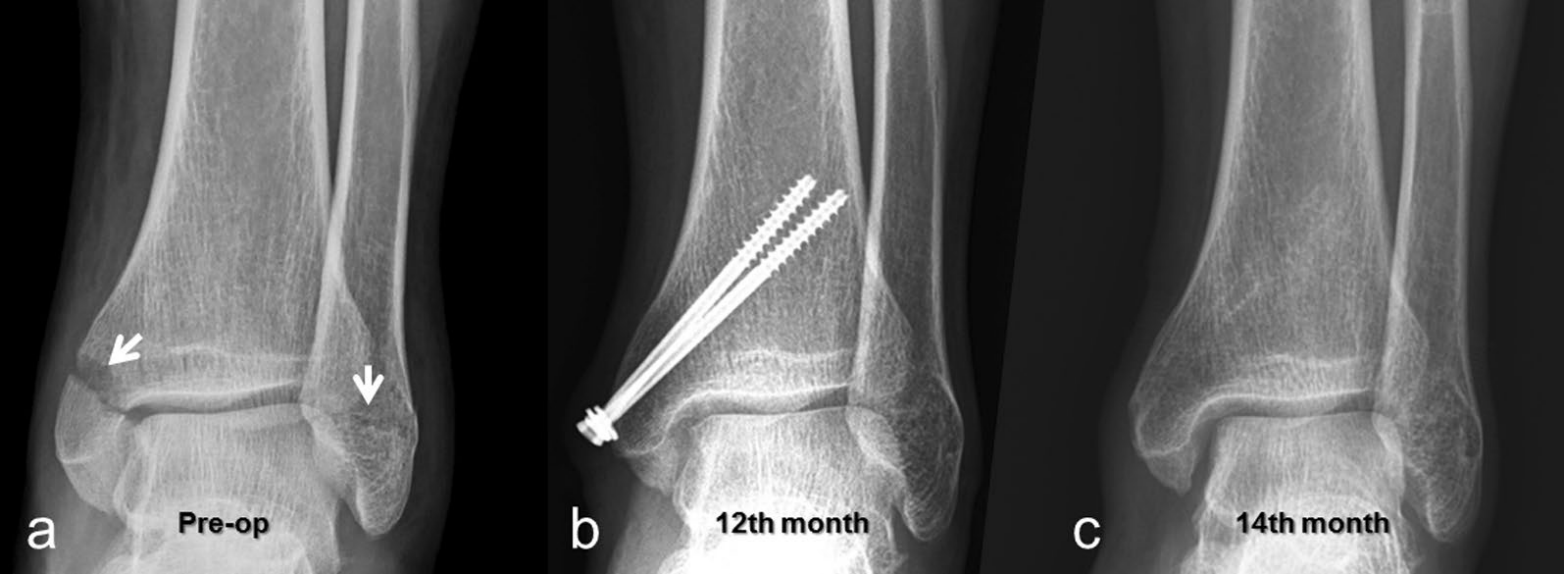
a Preoperative anteroposterior (AP) ankle radiograph of 44-year-old male patient with bimalleolar fracture (white arrows showing fractures); b At 12-month follow-up, fracture was united, but patient had pain over medial malleolus; c AP ankle radiograph 2 months after removal of screws
